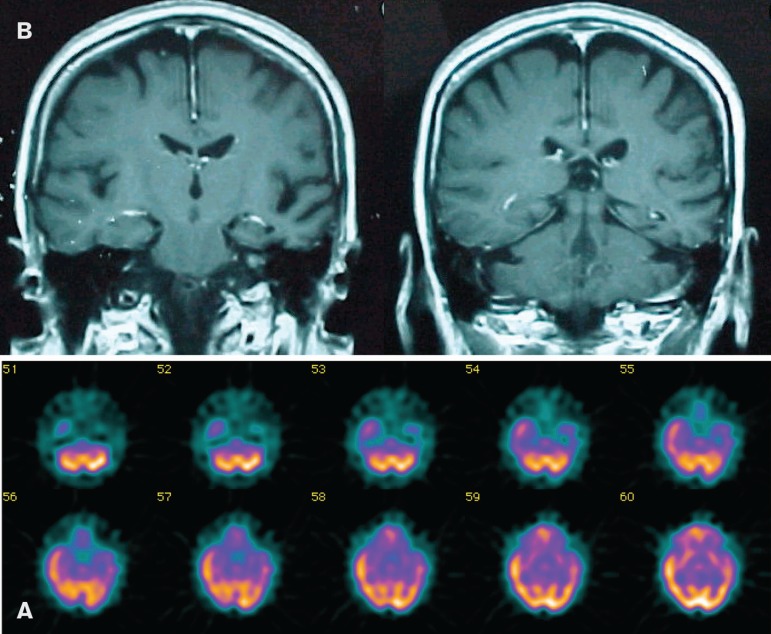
(A) Magnetic resonance imaging (MRI) showing atrophy of the left
